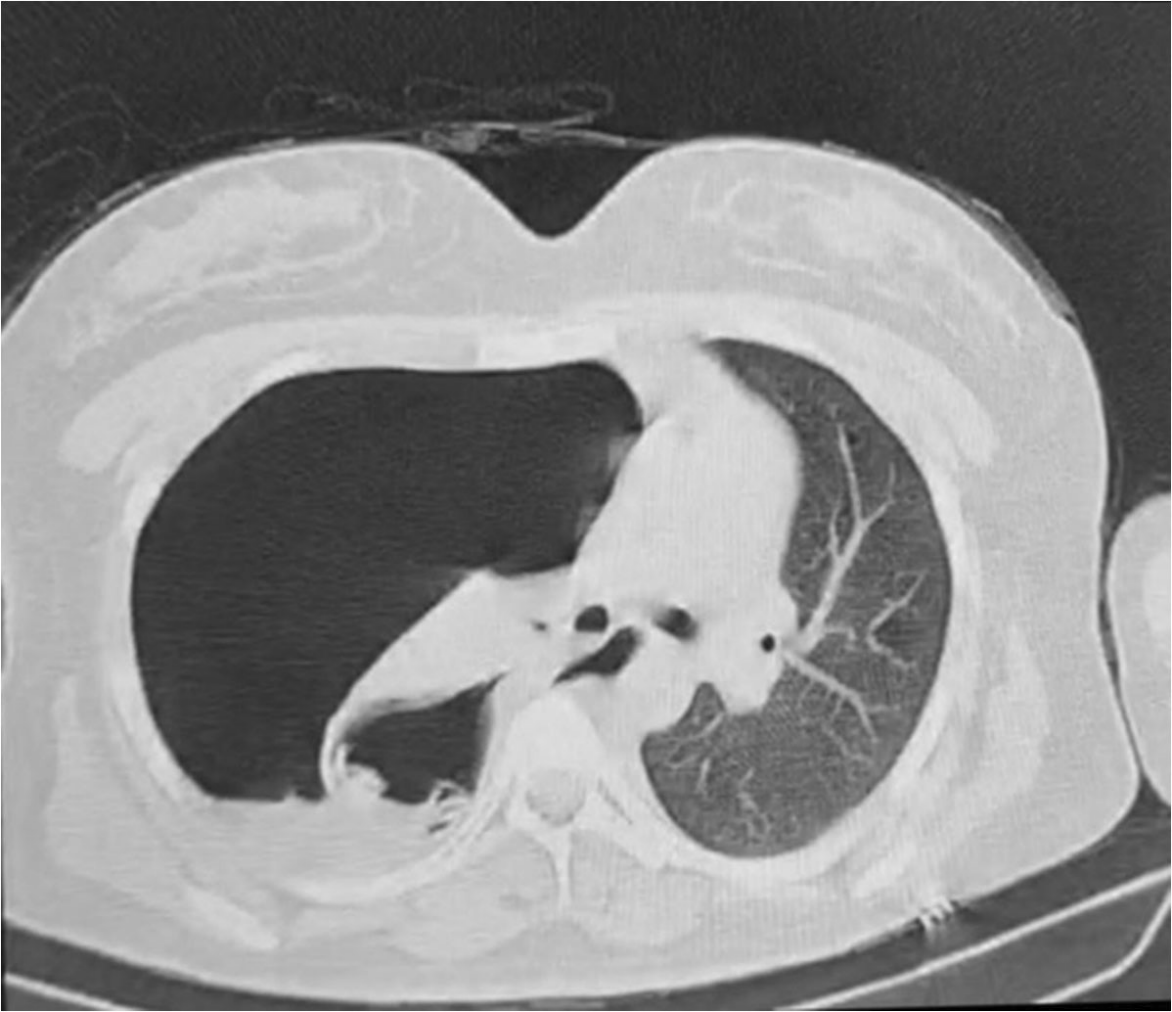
Chest CT scan of Case 2 indicating a mediastinal shift to the left side and floating membranes in the ruptured cyst on the right side.
